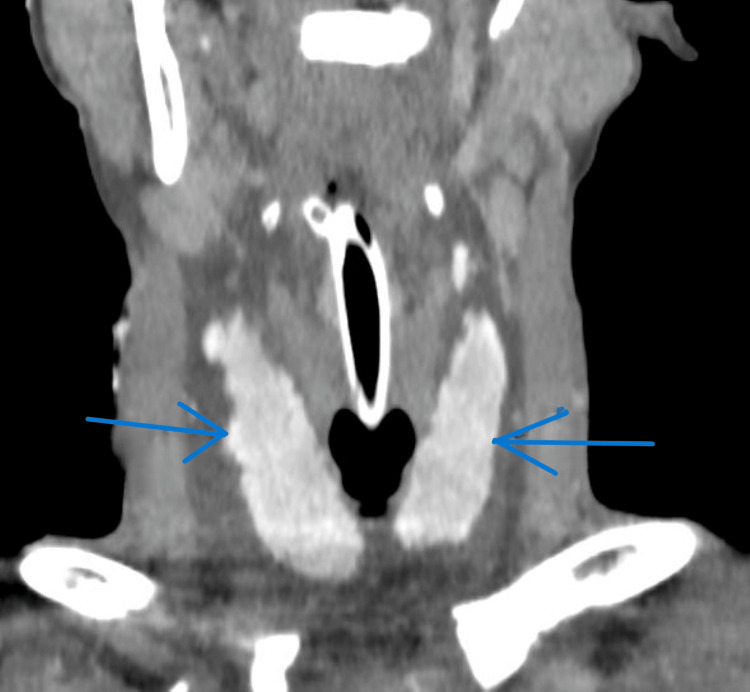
Coronal contrast-enhanced CT of the neck showing shock-related thyroid change. Contrast-enhanced computed tomography (CT) scan of the neck in coronal view (venous phase; slice thickness 5 mm; window/level 350/50 HU) showing diffuse symmetric enlargement of the thyroid gland with perithyroidal low-density fluid (blue arrows) consistent with shock-related thyroid change. The thyroid (T) gland demonstrates intact margins and homogeneous enhancement with irregular contours but without evidence of laceration or hematoma. HU: Hounsfield unit
